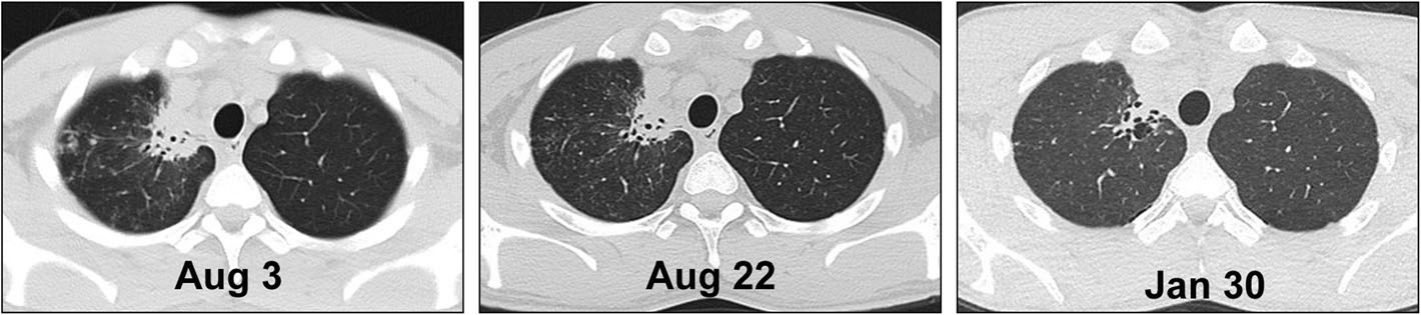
Follow-up CT scan of the lungs on August 22, 2018 and January 30, 2019. Absorbed exudation of the inflammatory lesion as the treatment continues, leaving small cavities and organic foci
